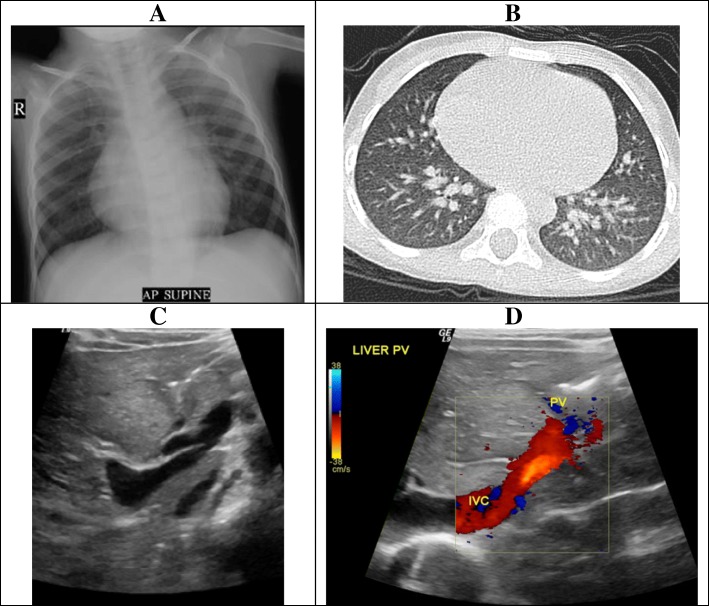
Representative of the pre-operative imaging. a Chest radiograph showing mild cardiomegaly with increased bronchovascular markings. b Contrast-enhanced axial chest computed tomography image showing normal lung parenchyma with a prominent pulmonary vasculature. c Ultrasound of the abdomen showing dilated inferior vena cava. d Doppler ultrasound of the abdomen showing portal vein (PV) shunting into the inferior vena cava (IVC)
